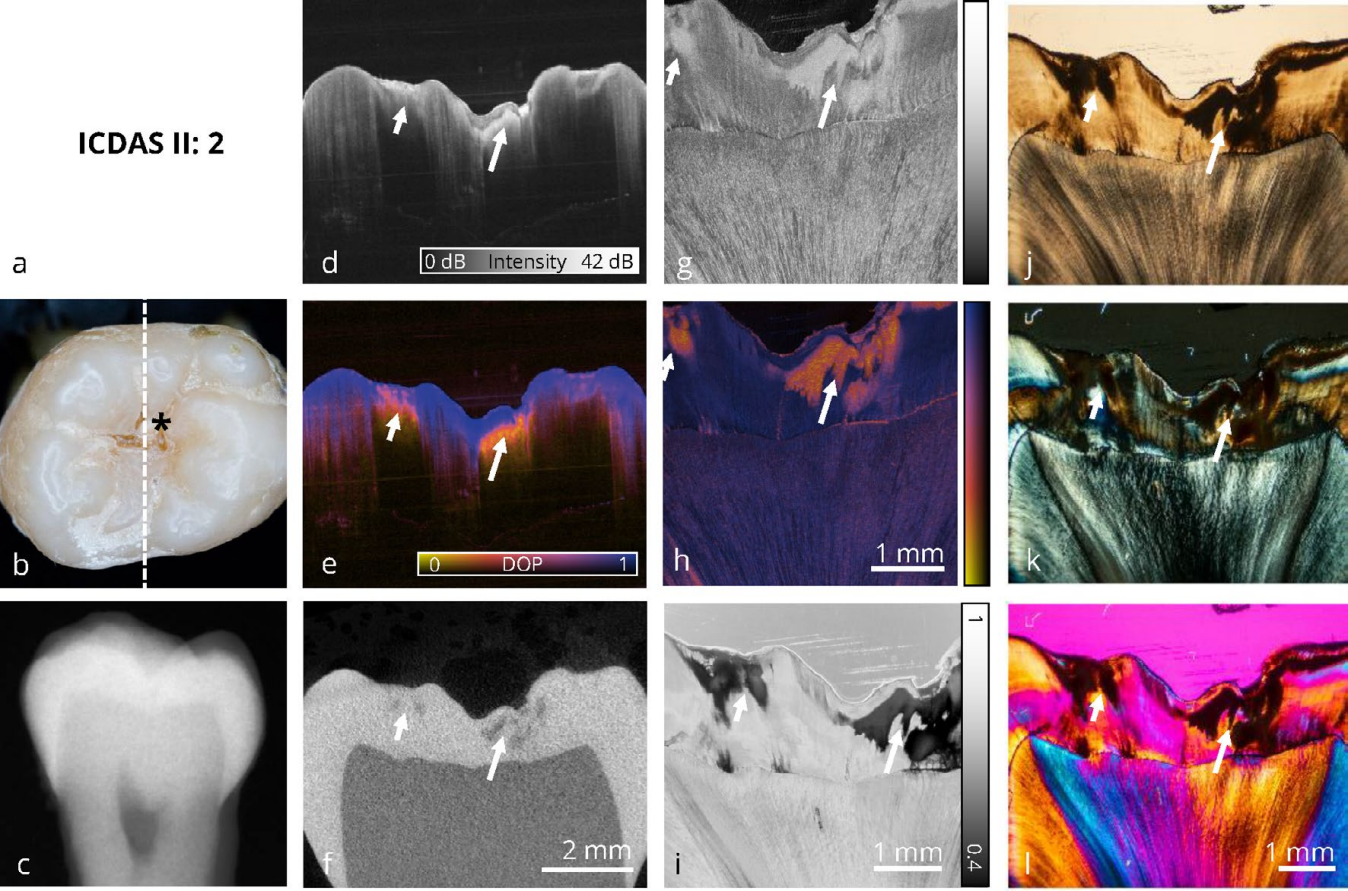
Collection of optical imaging data of an occlusal carious lesion in the same sectional plane. Calculus (*) is visible on the occlusal surface. Differentially, the structure on the occlusal surface may also represent a discoloration or an incipient carious lesion, while typical clinical signs of occlusal caries are absent. Thin sections reveal the presence of an initial enamel demineralization (→) that requires further monitoring. This early‐stage lesion is also clearly detected in the PS‐OCT volume, visible in both intensity (d) and DOP (e) imaging, where the DOP representation allows a more distinct visualization. PS‐OCT intensity imaging additionally reveals the presence of a surface deposit. In contrast, the digital bitewing x‐ray shows no abnormalities, showing the limitations of conventional radiographic diagnostics in the detection of early stage occlusal caries lesions. Refer to Figure 2 for a detailed enumeration.
